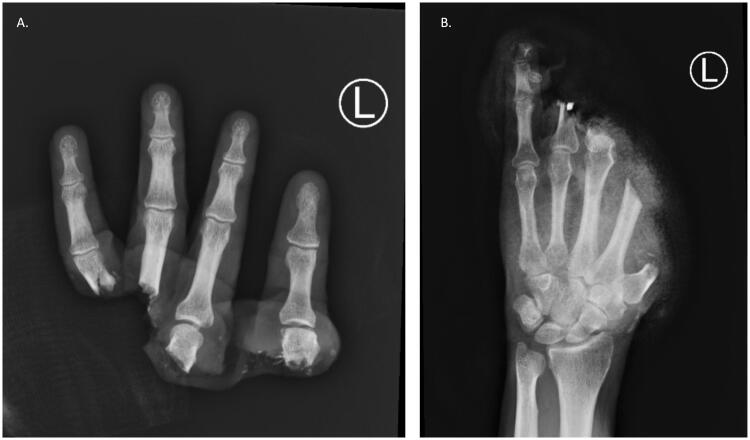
Left-hand and wrist radiography. Complete amputation of left-hand digits one through four with a sustained an open fracture-dislocation of the fifth finger on that side. First and second metacarpal fractures, open proximal phalangeal fractures of the middle and ring finger, and open fracture dislocation through the DIP joint of the small finger were found. There was trans metacarpal thumb and index amputation and a cross-proximal phalanx of middle and ring finger amputation.
